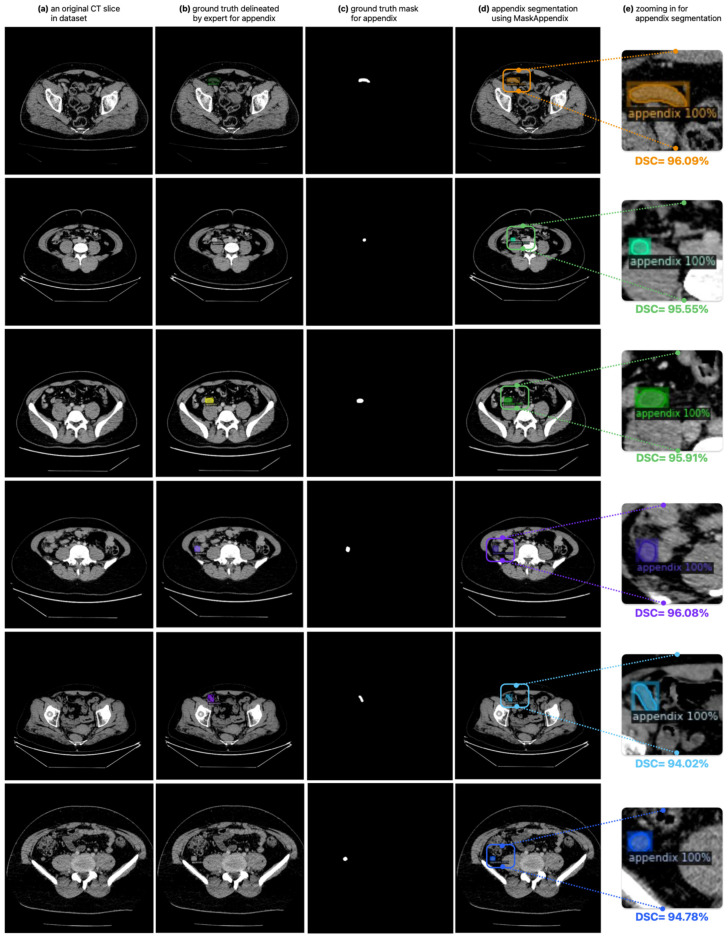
The successful automatic segmentation of the appendix in CT slices using the proposed MaskAppendix model.
